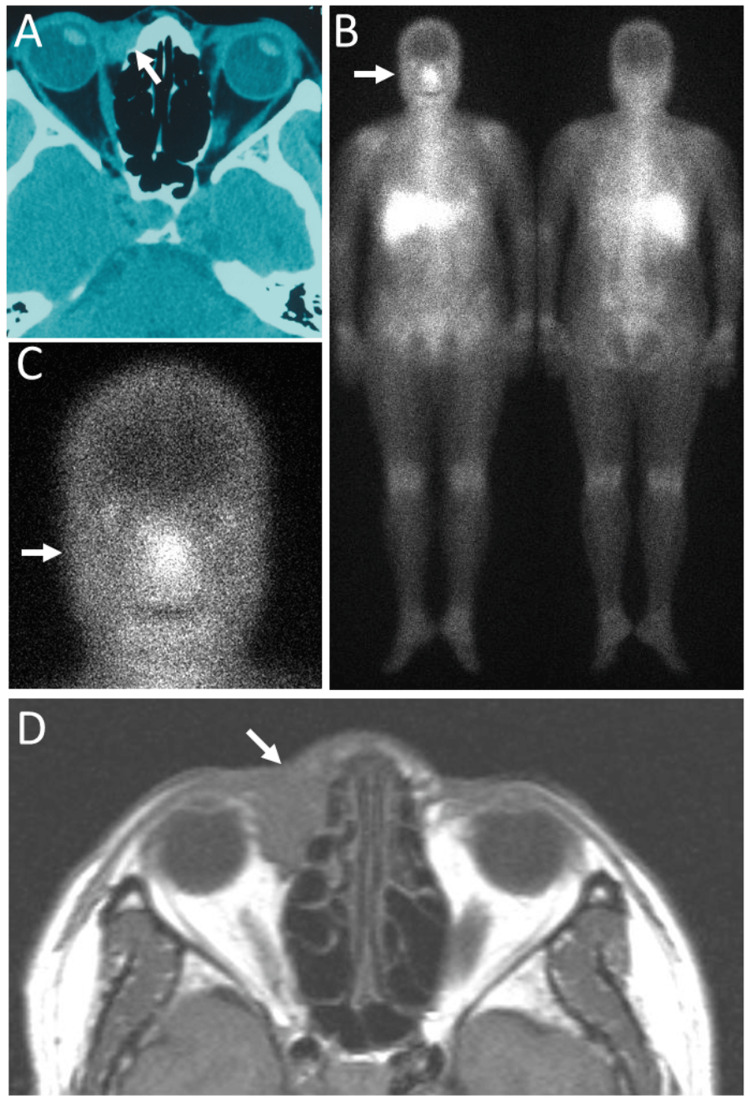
Computed tomography scan, gallium scintigraphy, and magnetic resonance imaging at age 54 years Computed tomography scan (A), showing right lacrimal sac mass (arrow) at age 54 years. After excisional biopsy to confirm diffuse large B-cell lymphoma, gallium scintigraphy (B, C), showing abnormal high uptake only at the right lacrimal sac area (arrows). T1-weighted magnetic resonance imaging (D), showing homogeneous right lacrimal sac mass (arrow).
